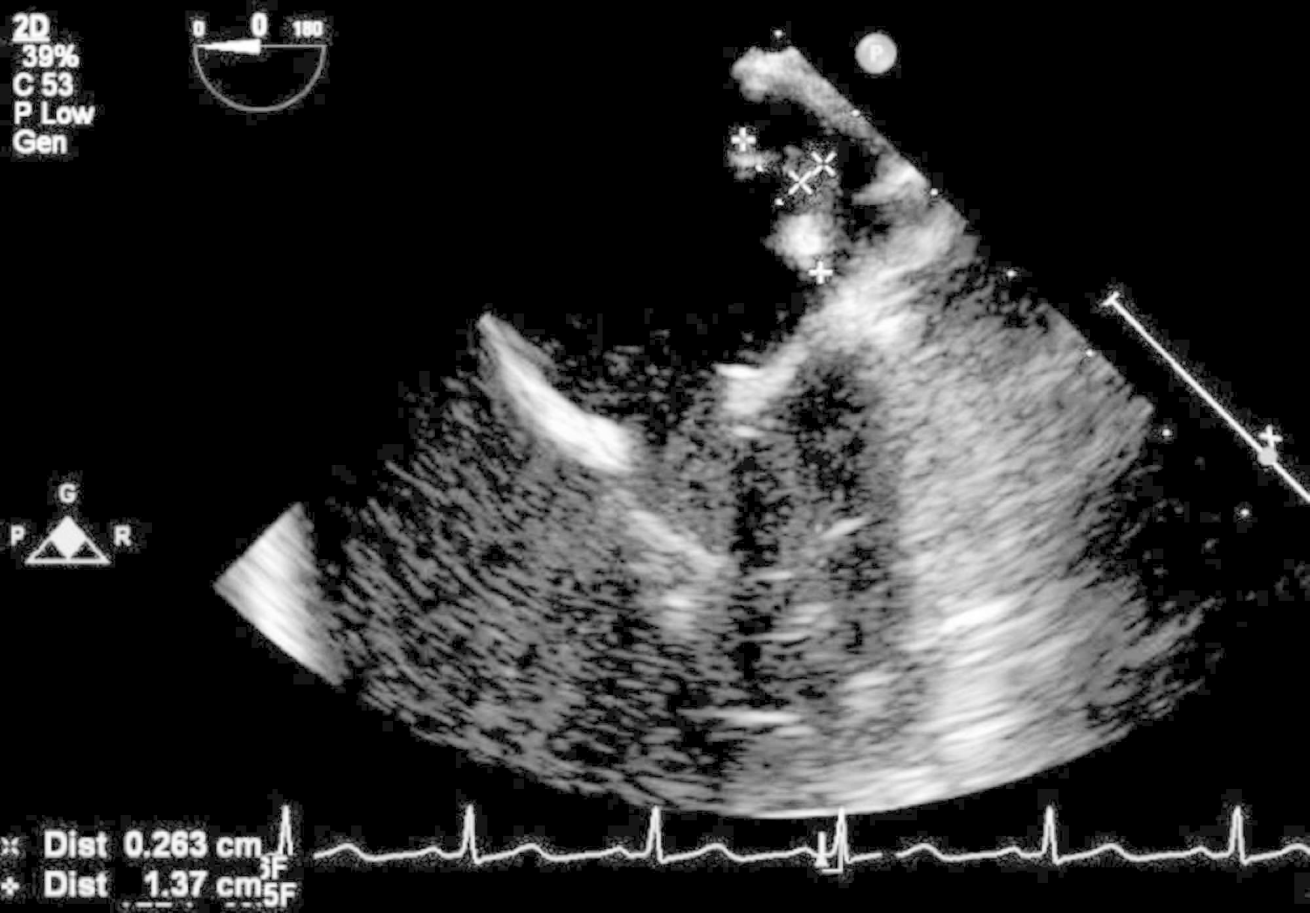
Transesophageal echocardiogram showing 1.37 x 0.263 cm pedunculated mass on the posterior wall of the left atrium, indicated by the caliper marks for measuring it, in the upper portion of the image
